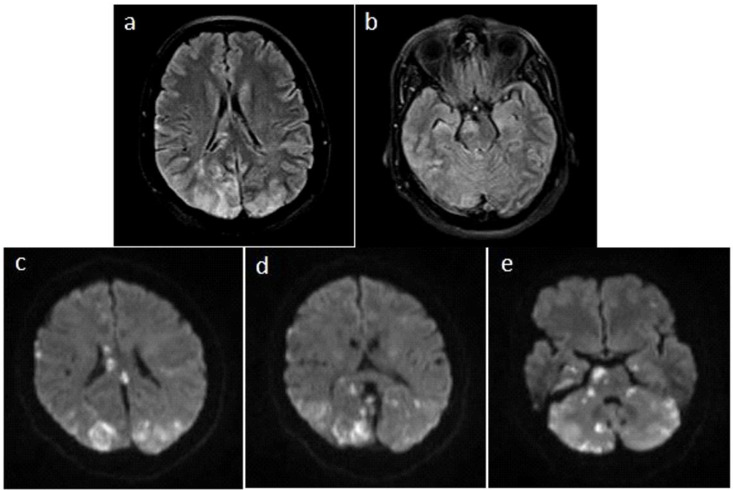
Axial ﬂuid-attenuated inversion recovery (FLAIR) (a, b) and diffusion-weighted (c, d, e) magnetic resonance images 2 days after presentation indicates increased signal intensity in parietooccipital lobes and pons
